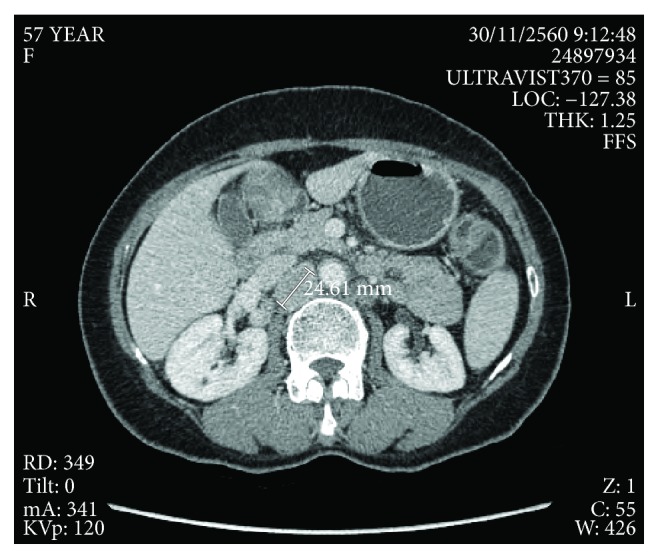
The following CT scan revealed a marked decrease in size of intra-abdominal lymph nodes.
